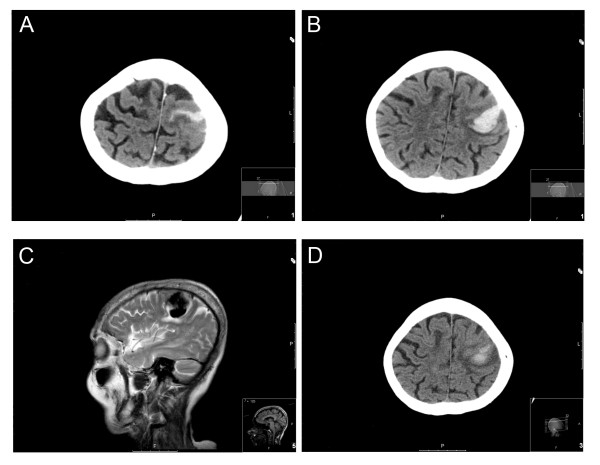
Localisation of haemorrhagic event. (a, b) CT radiographic imaging demonstrating a peripheral acute haematoma involving the left periolandic cortices extending over the left lateral cerebral convexity. (c) T2-weighted MRI demonstrating peripherally located haematoma within the left parietal lobe with surrounding oedema and mild compression of the precentral gyrus. (d) CT radiographic imaging 7 days later demonstrating maturing left precentral haematoma.
